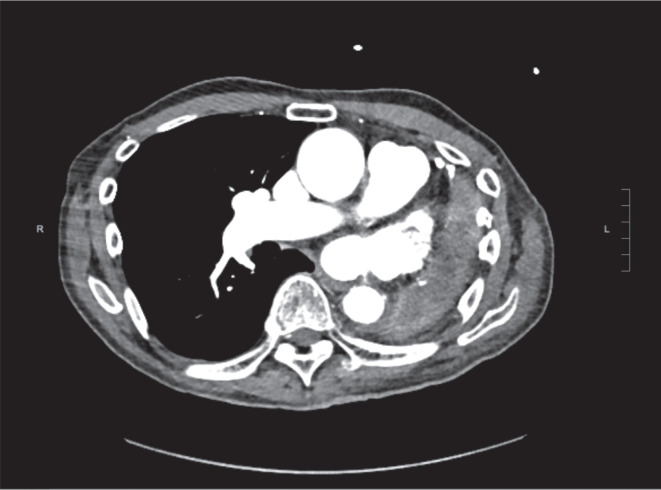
Cardiac CT scan demonstrating a left mediastinal shift in the patient.
